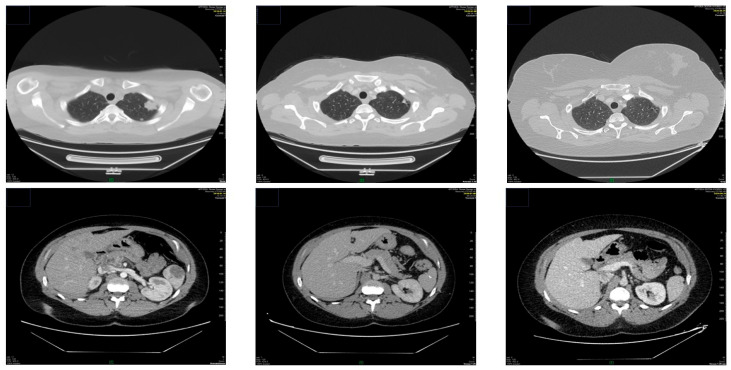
We can see the CT image of the lung metastasis and its complete regression (upper line) and the splenic metastasis and its complete regression. GE Optima^TM^ 660 (GE Health Care, 500 West Monroe Street, Chicago, IL 60661, USA) was used.
